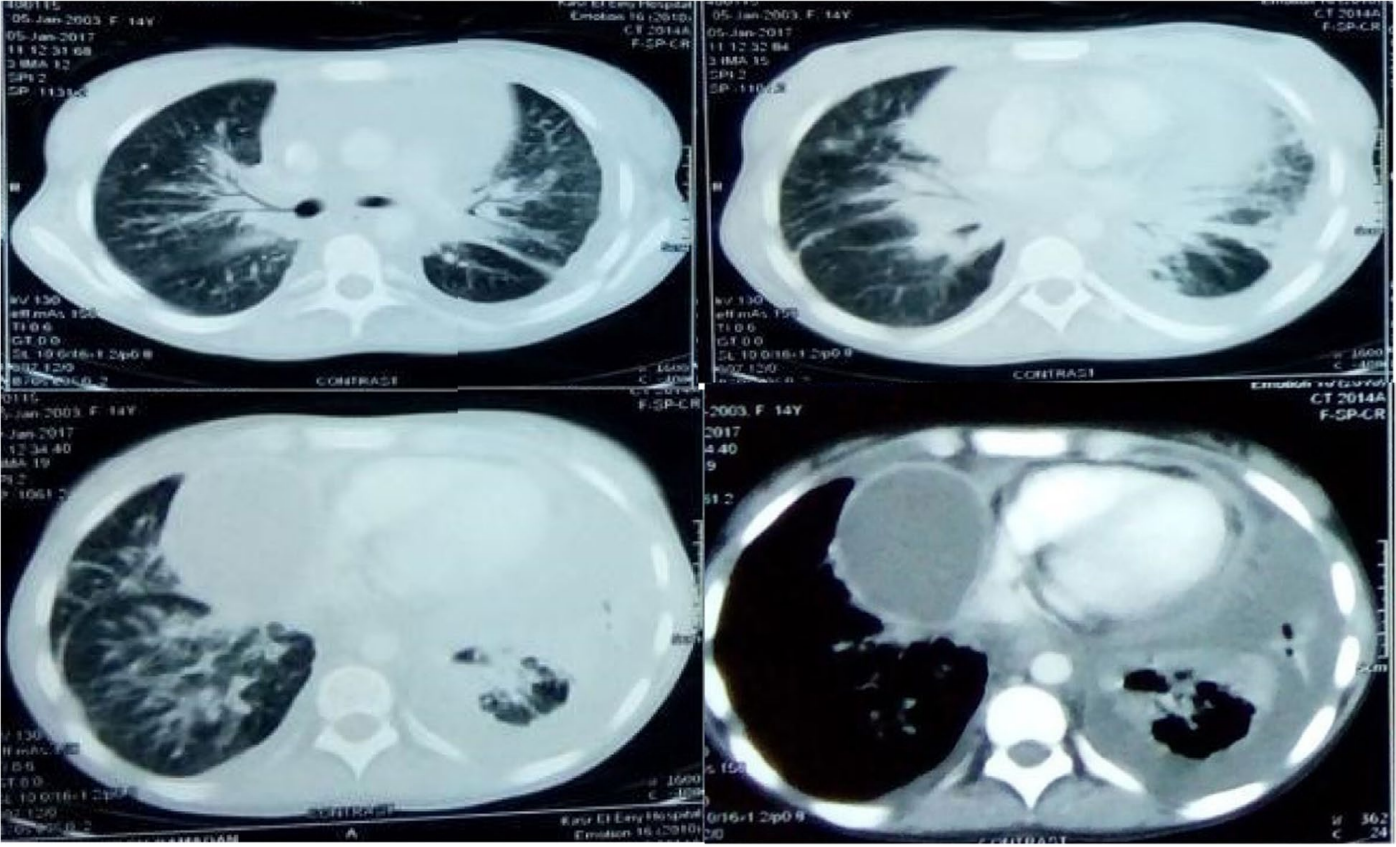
CT chest: lung window showed consolidation with air bronchogram in lung midzone and lower lobes. Mediastinal window (lower right) showed massive pericardial effusion, encysted effusion
